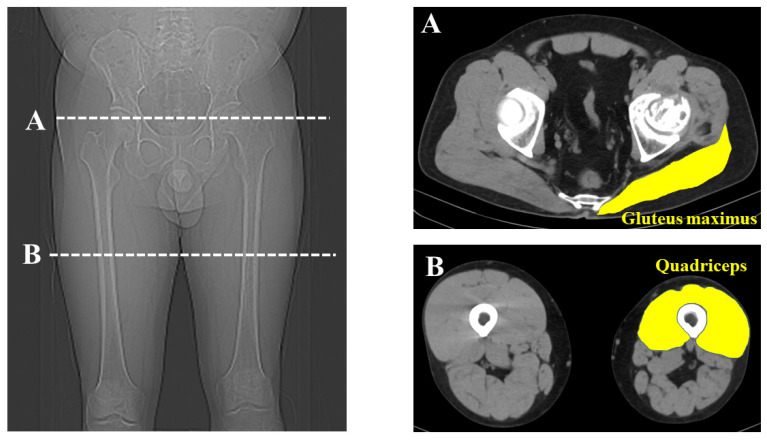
Measurement of muscle volume and Hounsfield Units. Cross-sectional measurement of the gluteal maximus and quadriceps was performed using computed tomography images at the greater trochanter (A) and the middle of the thigh (B).
